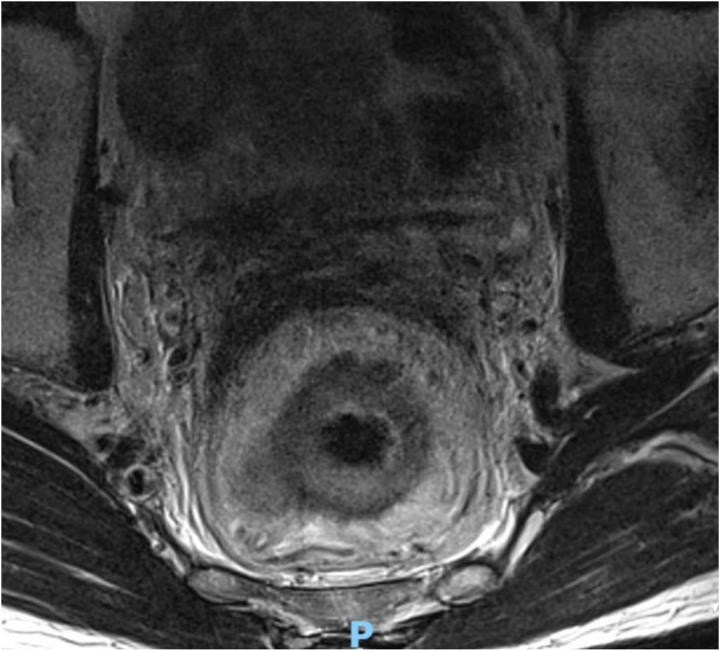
Magnetic resonance image of the rectum demonstrating annular thickening of the mid to upper rectum starting 7.5cm above the anal verge with linear T2 signal suggesting mucinous component
